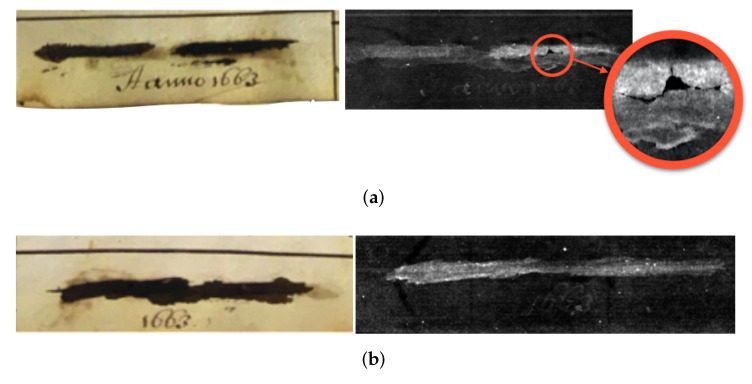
Two examples of the false positives with photographs of the scribblings on the left, and processed radiographs on the right. The top set in (a) indicate a partially torn page, and the bottom set in (b) show no detectable calligraphy in the post-processed image.
